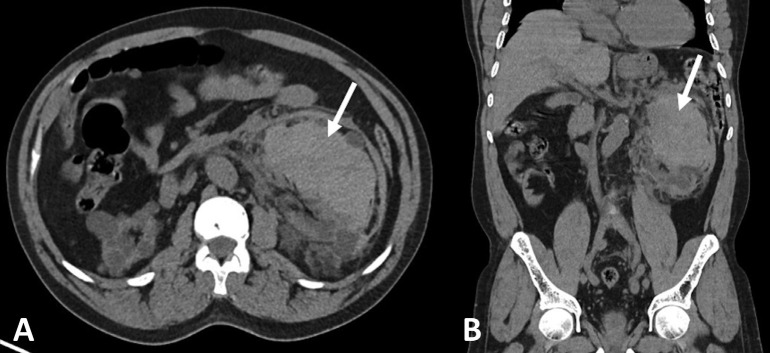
Contrast-enhanced CT showing left renal and perirenal hematoma (white
